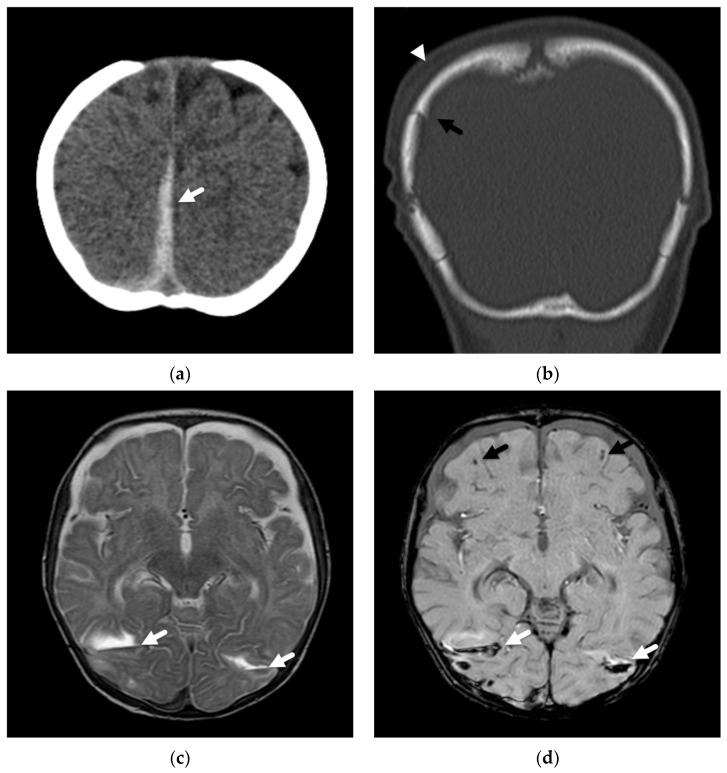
Axonal injury and lacerations/contusional tears in a 4-month-old male presenting with seizure and lethargy. (a) Initial axial CT image demonstrates right parafalcine high-attenuation subdural hemorrhage (white arrow); (b) coronal reconstruction in bone algorithm demonstrates a right parietal bone fracture (black arrow) with overlying soft tissue swelling (white arrowhead); (c) axial T2-weighted MR image on day 4 demonstrates bilateral lacerations/contusional tears with fluid-hemorrhagic levels near the temporal-occipital lobe junctions (white arrows); (d) axial susceptibility-weighted image demonstrates layering hemorrhage in lacerations/contusional tears (white arrows) and axonal injury near the cortical-white matter junction in each frontal lobe (black arrows).
